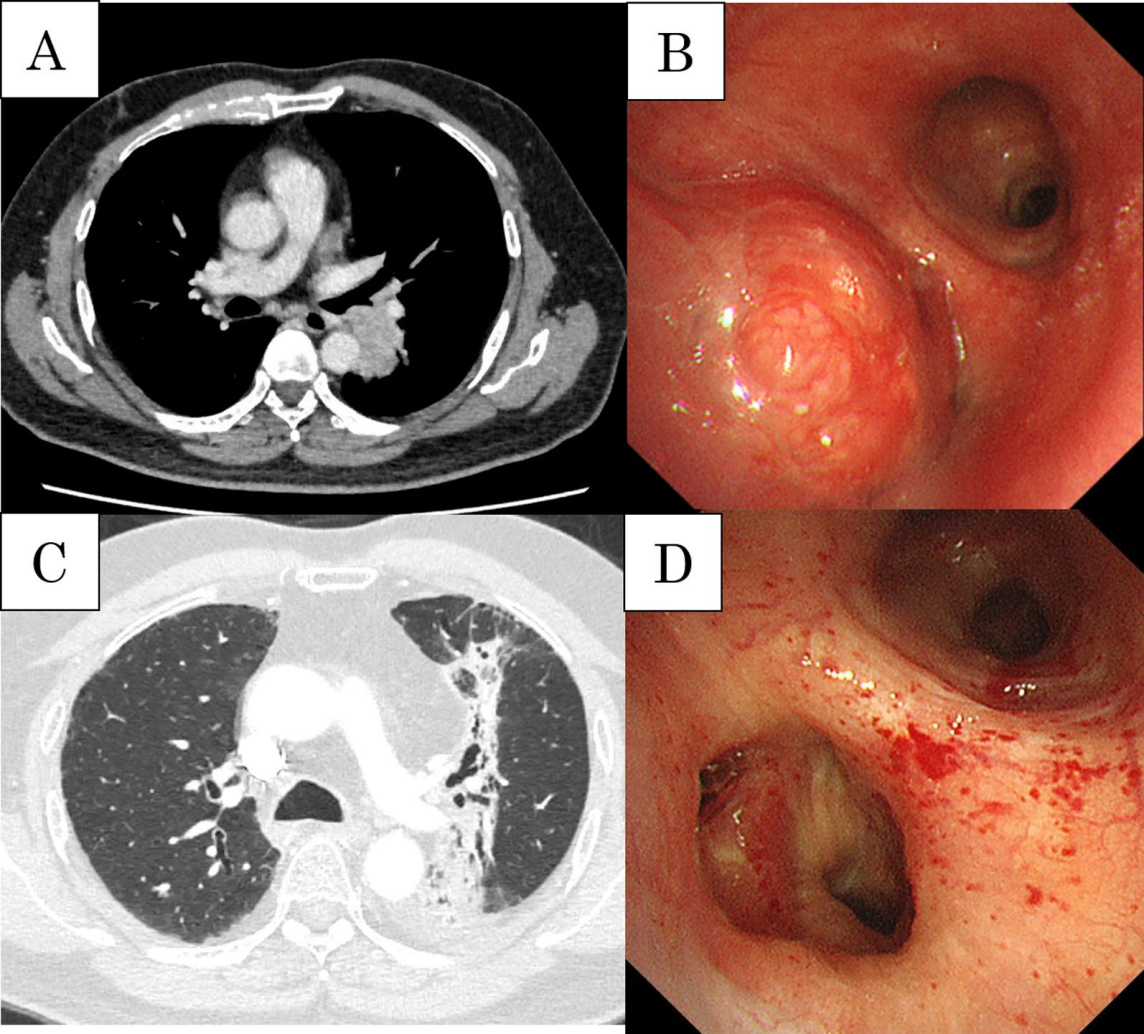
Preoperative CT and bronchoscopic findings. A Initial CT showing squamous cancer in the left lower lung lobe. B The tumor protruding from the left lower bronchus and invading the left main bronchus. C CT showing moderate radiation pneumonitis after induction chemoradiation therapy. D Tumor regression after preoperative therapy shown by the appearance of the left lower bronchus
